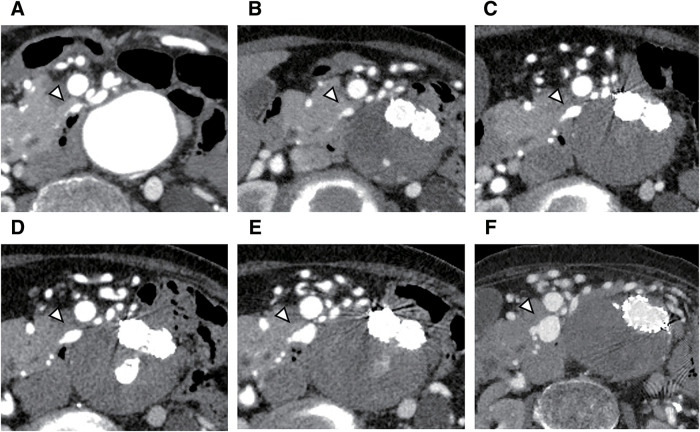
Serial computed tomography images demonstrating progressive enlargement of the (IPDAA, white arrowheads). (A) Before CA embolization, the IPDAA measured 2.5 mm. (B) Immediately after CA embolization, the aneurysm size remained unchanged (2.5 mm). (C) At 8 months post-embolization, the aneurysm enlarged to 5.0 mm. (D) At 2 years, the aneurysm enlarged to 6.5 mm. (E) At 5 years, the aneurysm size increased to 8.9 mm. (F) At 10 years, the aneurysm markedly enlarged to 15.0 mm. IPDAA: inferior pancreaticoduodenal artery aneurysm; CA: celiac artery
